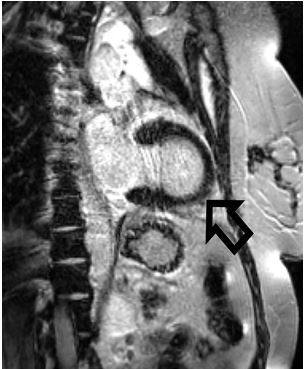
Sagittal magnetic resonance imaging showing apical ballooning of left ventricle.
